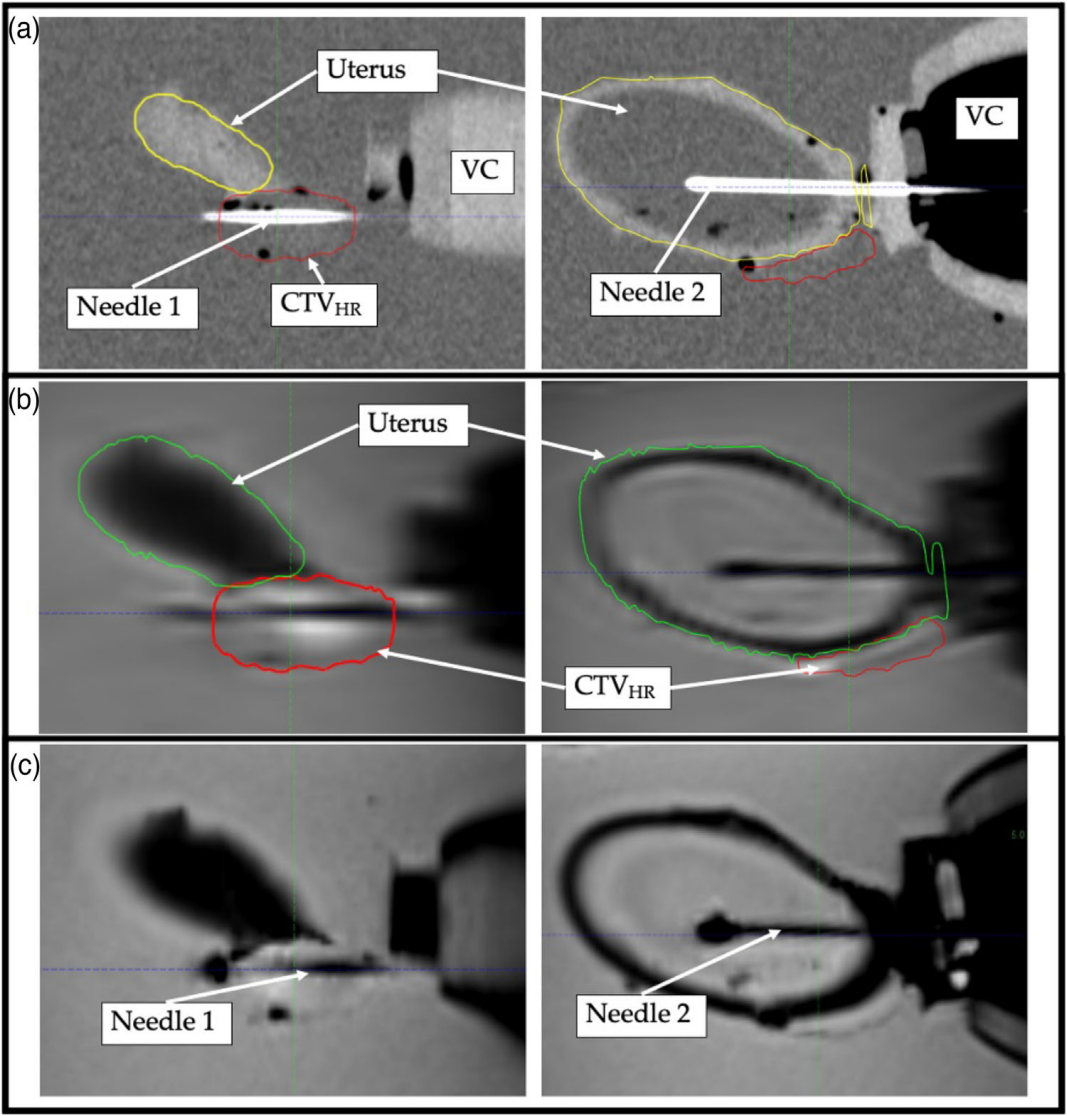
Two sagittal planes showing the pelvic anatomy and inserted needles: (a) CT; (b) T2‐weighted MR; (c) T1‐weighted MRI. VC represents the vaginal canal.
